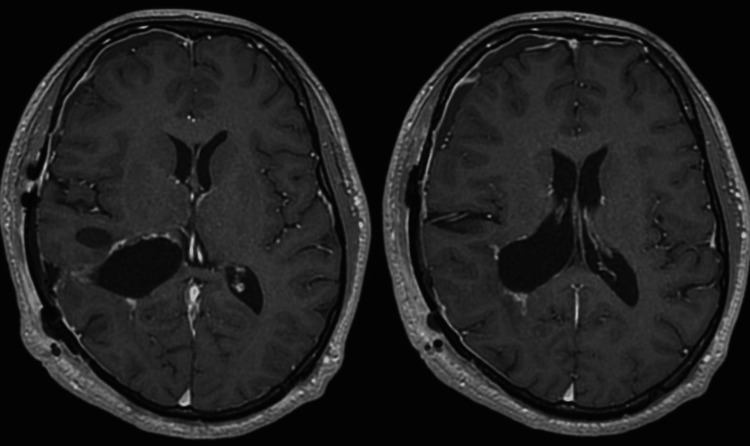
Postoperative MRI after second surgery showing gross total removal of the tumor Contrast-enhanced T1-weighted image showing the adequate removal of the tumor MRI: magnetic resonance imaging
